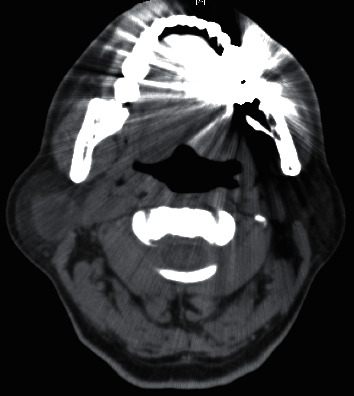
CT with contrast revealed an enhancing multilocular cystic lesion in the right parotid gland.
